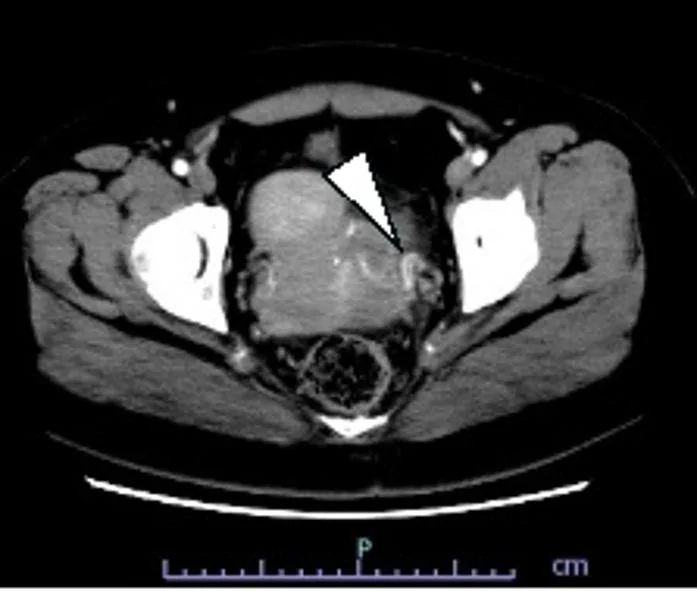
Contrast-enhanced CT demonstrates the ovarian artery and vein in a spiral shape, termed the whirlpool sign, suggesting that the ovarian vessels are twisted (arrowhead)
